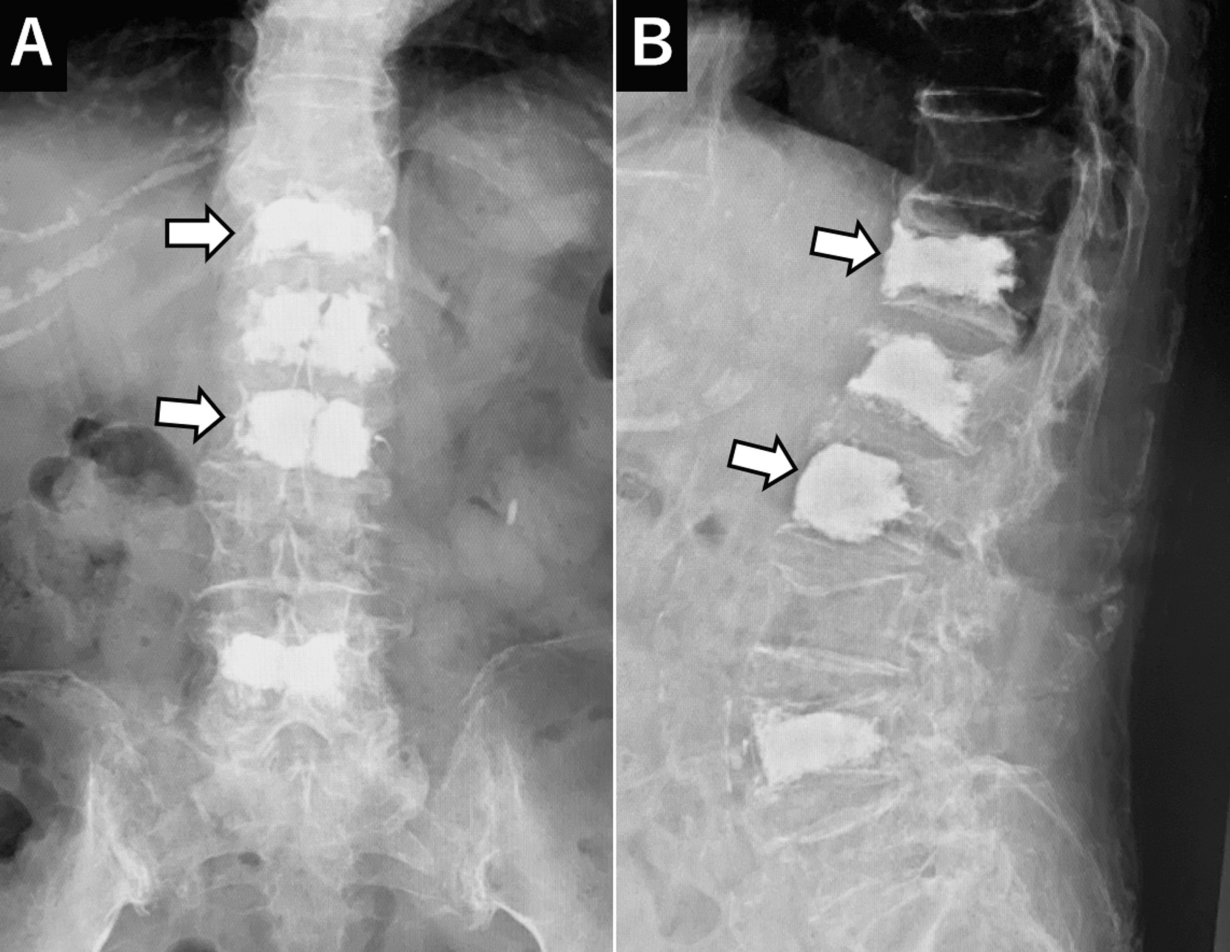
Post-second BKP X-ray An X-ray taken after the second balloon kyphoplasty (BKP) shows bone cement in Th12 and L2 (arrows).
